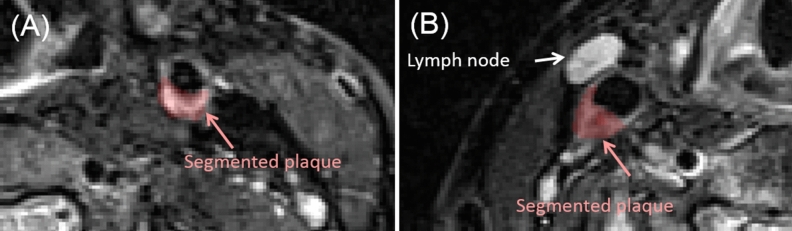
Examples of plaque delineations. (A) Vulnerable atherosclerotic plaques were identified and segmented on T2-TSE MR images. (B) Lymph nodes with non-negligible [^68^Ga]Ga-Pentixafor uptake located close to the segmented plaques were also included in the PVC evaluation
